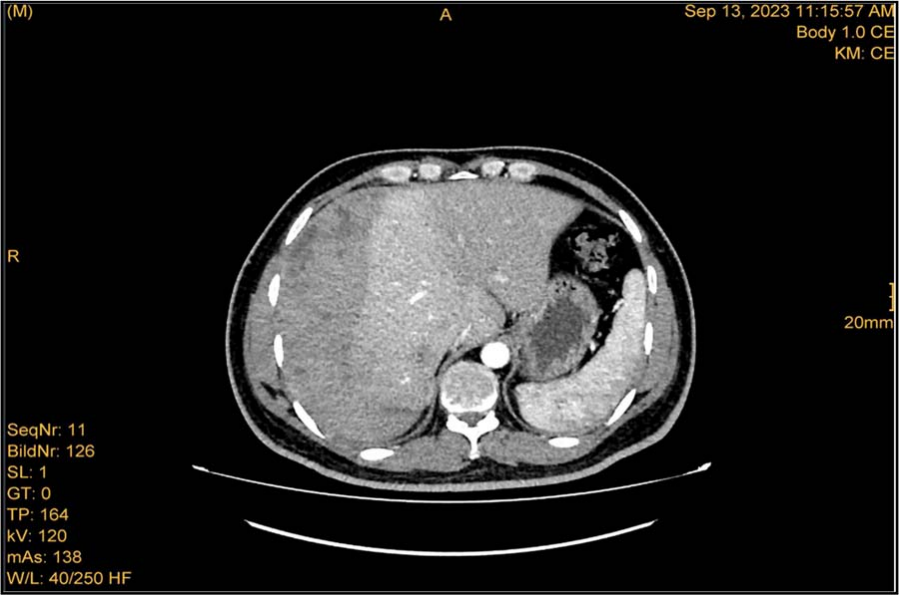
Enhanced CT scan utilizing bleeding protocol. Status post-LC identified significant ISH with internal hyperdensity measuring 7.1 × 19 × 21 cm, in its transverse, anteroposterior, and craniocaudal diameters, respectively. Adjacent hyperemic liver parenchyma with intraparenchymal hematoma noted at segment 5, measured 4.7 × 4 × 4.7 cm. Intraparenchymal hypodensities noted at segments 8 and 4b, likely represent hepatic contusions and lacerations. No evidence of active contrast extravasation.
